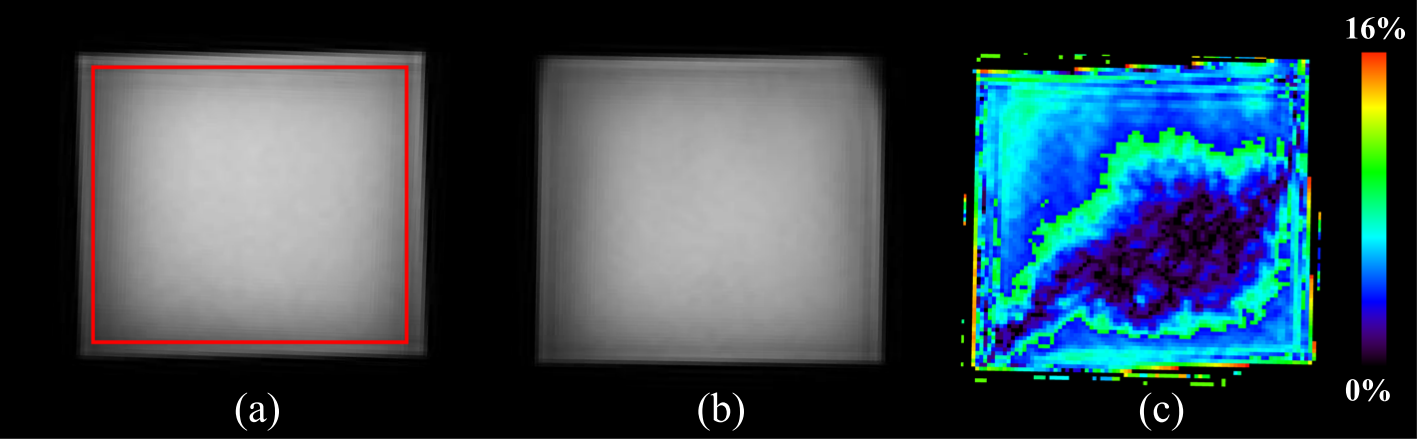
Dixon MR images with no DP-PET (a) and DP-PET inserted (b), and c is the result of subtracting a and b
